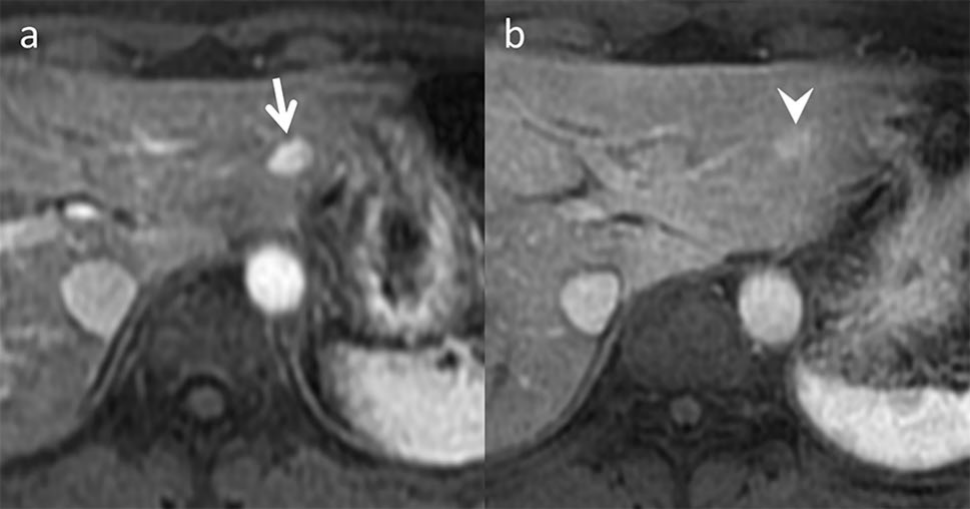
Hepatocellular adenoma in an asymptomatic 30-year-old woman, who was taking oral contraceptives. a Arterial phase of gadoxetic acid-enhanced magnetic resonance imaging (MRI) reveals a hyper-vascular lesion (9 mm in diameter) in segment 2 in the liver (arrow). b This lesion had shrunk (6 mm in diameter) in the arterial phase of gadoxetic acid-enhanced MRI after oral contraceptives were discontinued (arrowhead). Hepatocellular carcinoma is raised as a differential on imaging; however, it can be differentiated by age and the absence of chronic liver disease
